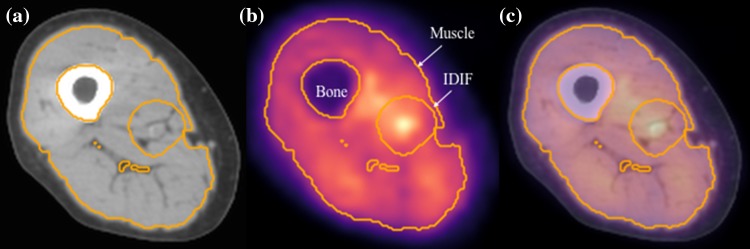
Images from Subject 004. CT (a) and PET (b) images corresponding to central mid-thigh cross section of thigh volume. The fused PET/CT image is shown in (c). The tissue activity curve (TAC) was computed from the region encompassing the total thigh muscle volume, but excluding bone and the region used to determine the image-derived input function (IDIF). These regions are superimposed in gold pixels on all three images
